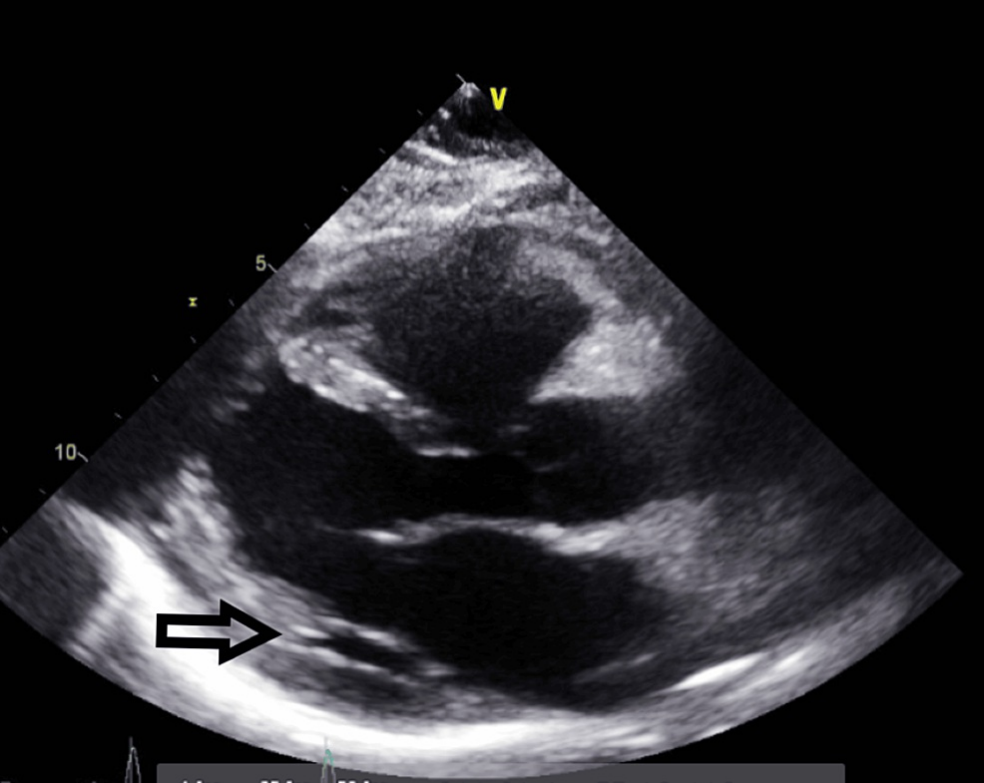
Transthoracic echocardiography - image 1 Transthoracic echocardiography on admission; parasternal long axis view shows minimal pericardial effusion
